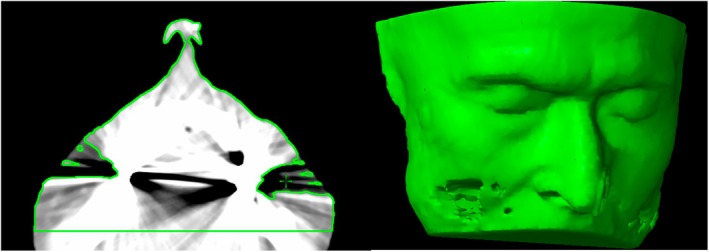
CT scans that have artefact from teeth fillings (left) and the effect on the 3D print (right).
